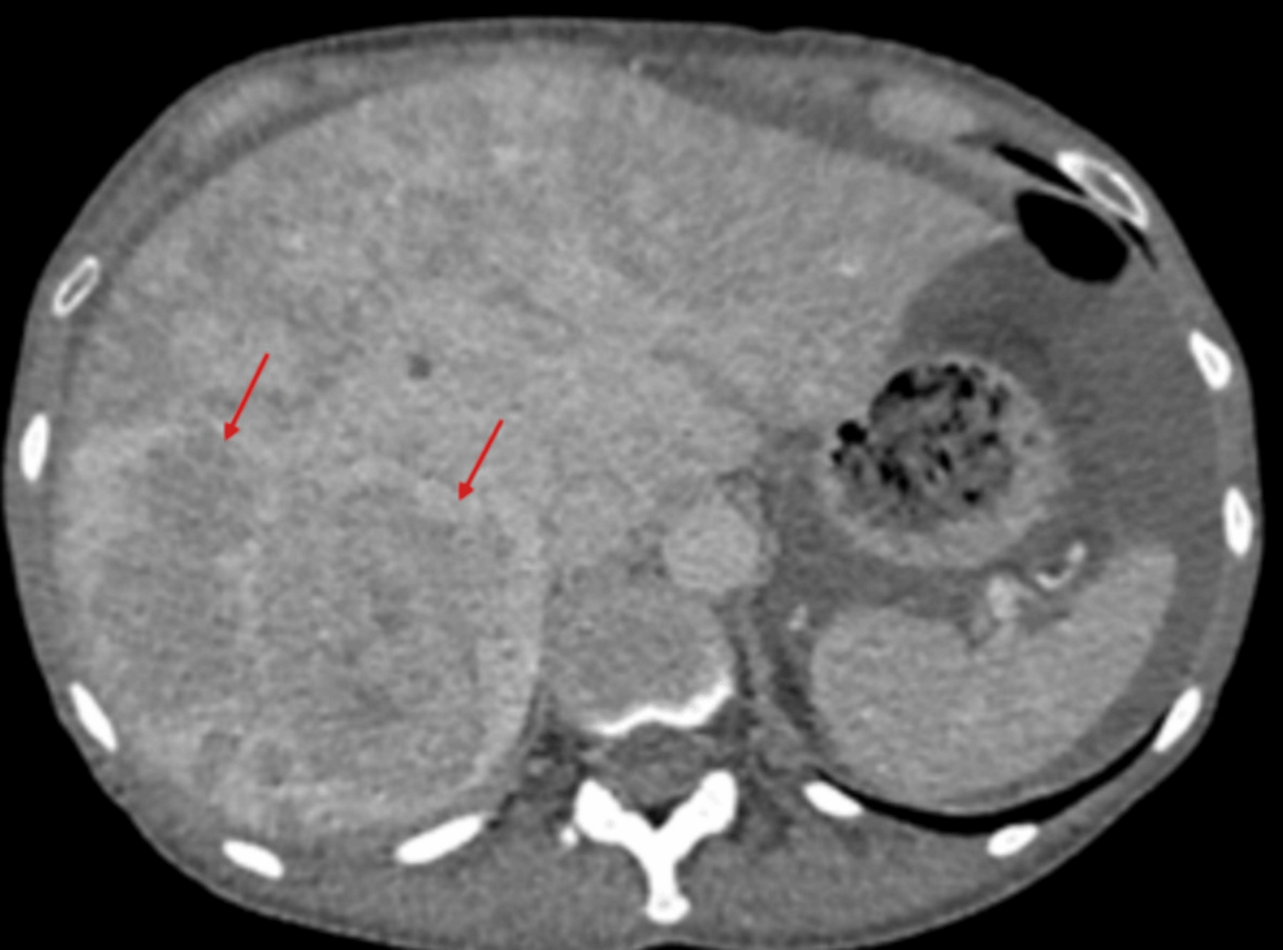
Repeat CT of the thorax/abdomen/pelvis on 7 February 2025 showing liver lesions (red arrows) with no evidence of gross interval changes.
